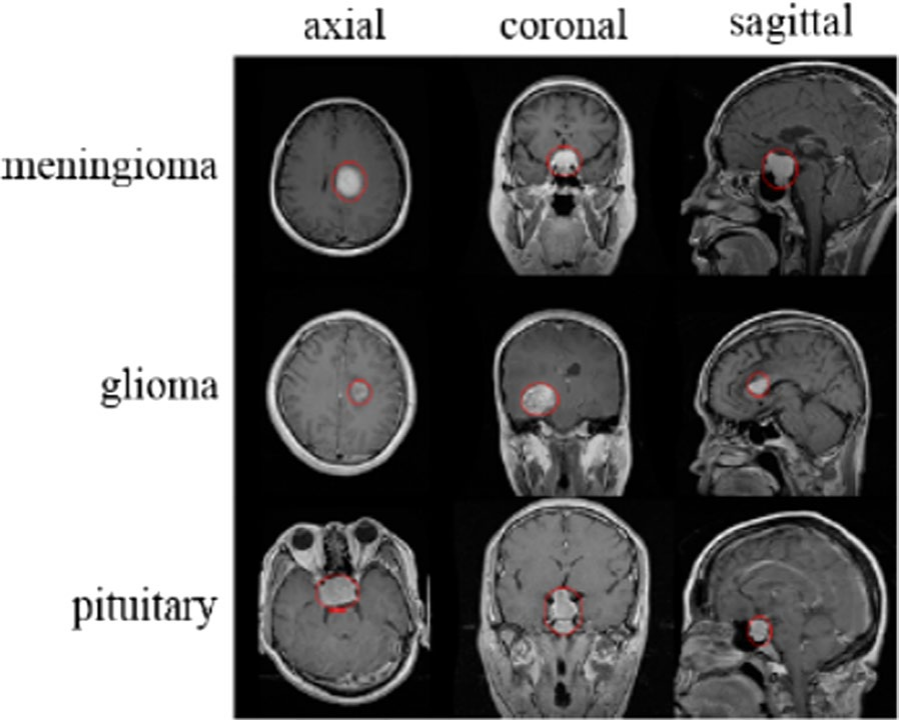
Description of normalized MRI images presenting diverse varieties of tumor in a different plane
